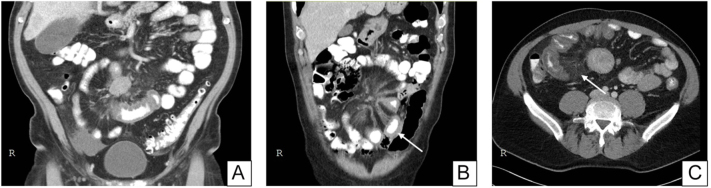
CT images of mesenteric fibrosis in SI-NET patients. (A and B) Coronal CT images with the characteristic ‘spokeswheel’ pattern of mesenteric fibrosis (MF), showing radiating soft tissue strands originating from a mesenteric metastasis. In panel (B), the white arrow highlights an oedematous, thickened intestinal wall caused by venous stasis, which may lead to mesenteric ischaemia. Additionally, on an axial CT image, MF is depicted in panel C as a ‘misty mesentery’, characterized by increased attenuation of the fat tissue. A full colour version of this figure is available at https://doi.org/10.1530/ERC-25-0116.
